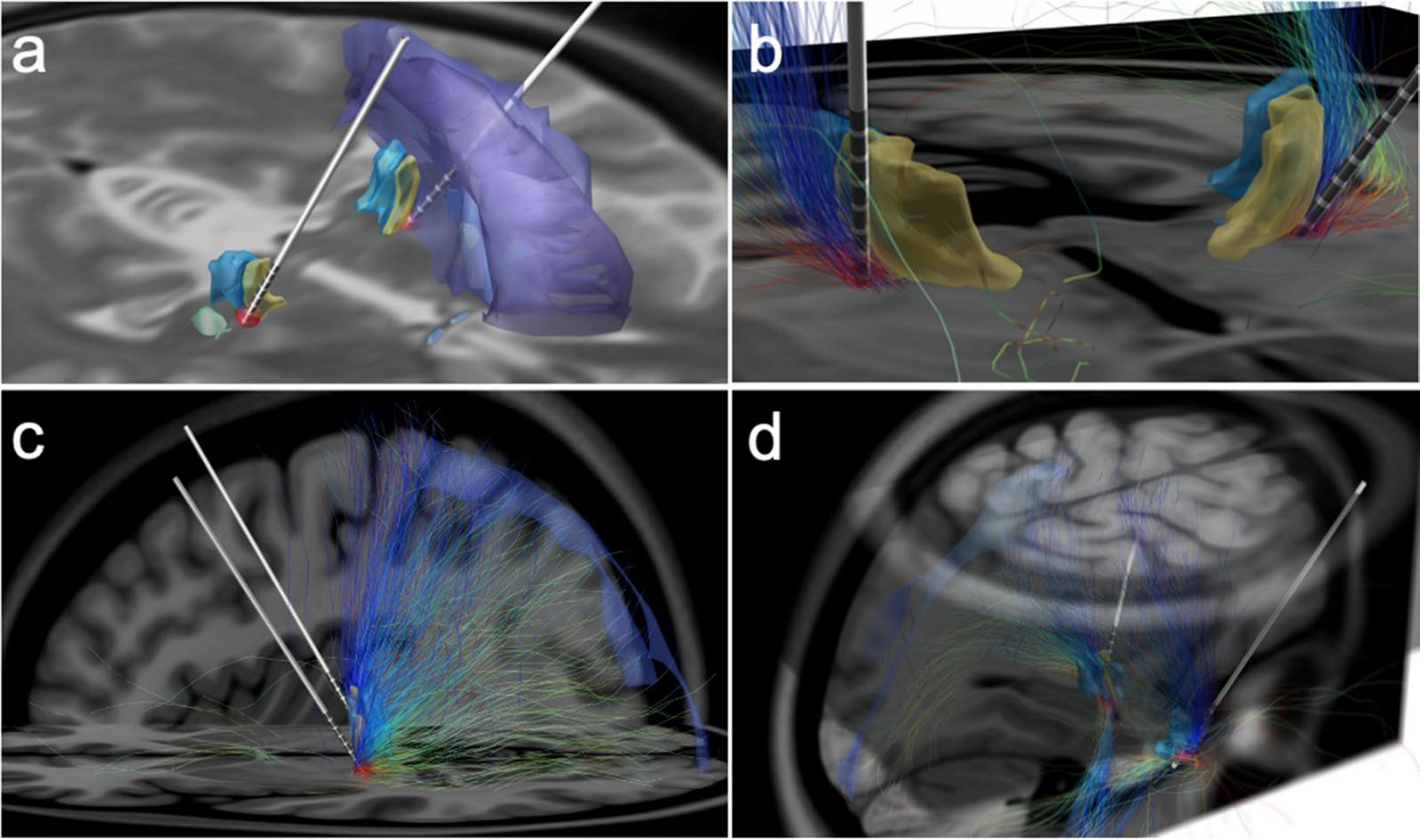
Perspective view of the estimated volume of tissue activated (VTA) in relation to the involved thalamic nuclei (a). Tractography (b) and connectivity study of the left hemisphere (c, d) revealing fibers passing through the VTA with cortical projection to the primary sensory cortex and the posterior cerebral quadrant (light blue area overlaid on normalized T1 MRI scan). VTA (red spheres), ventral posterolateral nucleus anterior (yellow); ventral posterolateral posterior (blue); medial lemniscus (light yellow)
